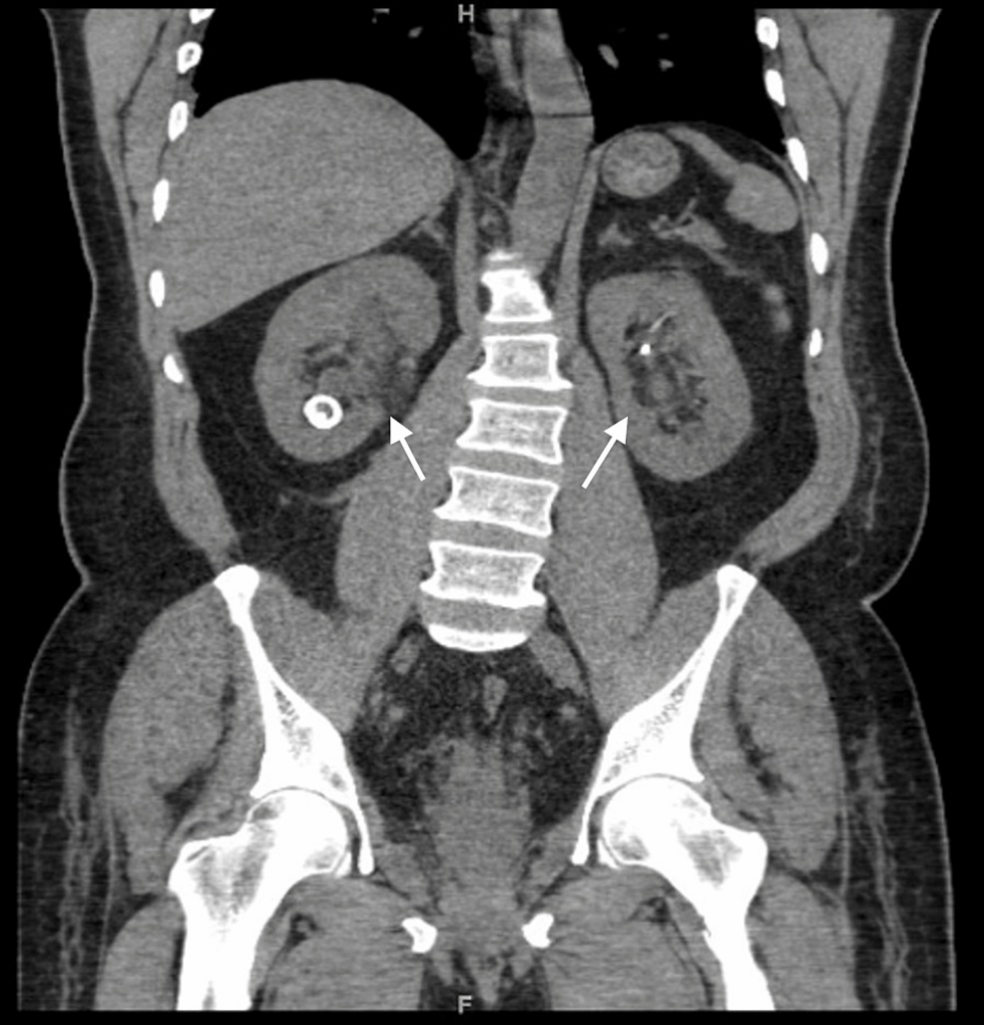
Post-operative CT image Marked improvement of bilateral hydronephrosis (white arrows) after ureteral reimplantation.
